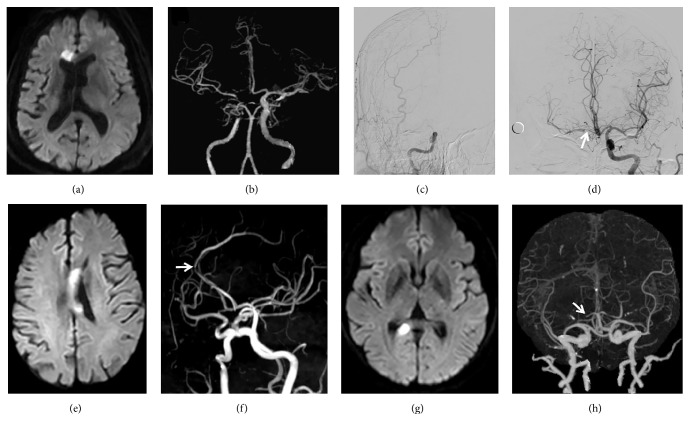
Infarctions at different sites of the corpus callosum and their affected vessels.* (a-d) Male, 69 years, left hemiparesis. Diffusion-weighted imaging (DWI) shows a new infarction of the right genu (a). Computed tomographic angiography (CTA) and digital subtraction angiography (DSA) reveal complete occlusion of the cavernous segment of the right internal carotid artery, collateral compensation from the left to the right anterior circulation via the anterior communicating artery, and severe stenosis of the A1 segment of right anterior cerebral artery (white arrow) (b-d). (e-f) Male, 75 years, right hemiplegia. DWI shows a new infarction of the left body (e). Magnetic resonance angiography (MRA) shows that the affected vessel is the severe stenotic pericallosal artery (white arrow) (f). (g-h) Female, 57 years, dizziness. DWI shows an isolated infarction of the right splenium (g). CTA shows that the P1 segment of right posterior cerebral artery has occluded (white arrow) (h).
